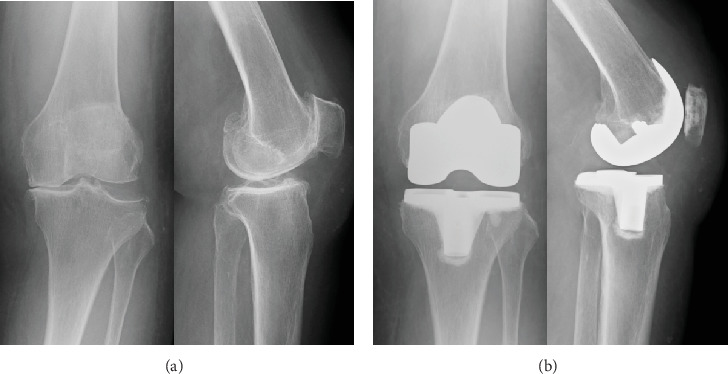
(a) Preoperative anteroposterior and lateral radiograph of the left knee. (b) Radiograph post-TKA.
